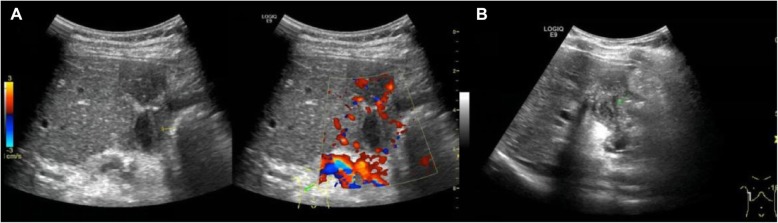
Color Doppler ultrasonography of an 8-year-old girl after Rex-bypass operation for CTPV. No blood flow signal was found in the bypass vessels, and thrombosis was observed. a 7 days after operation. b 6 months after operation
